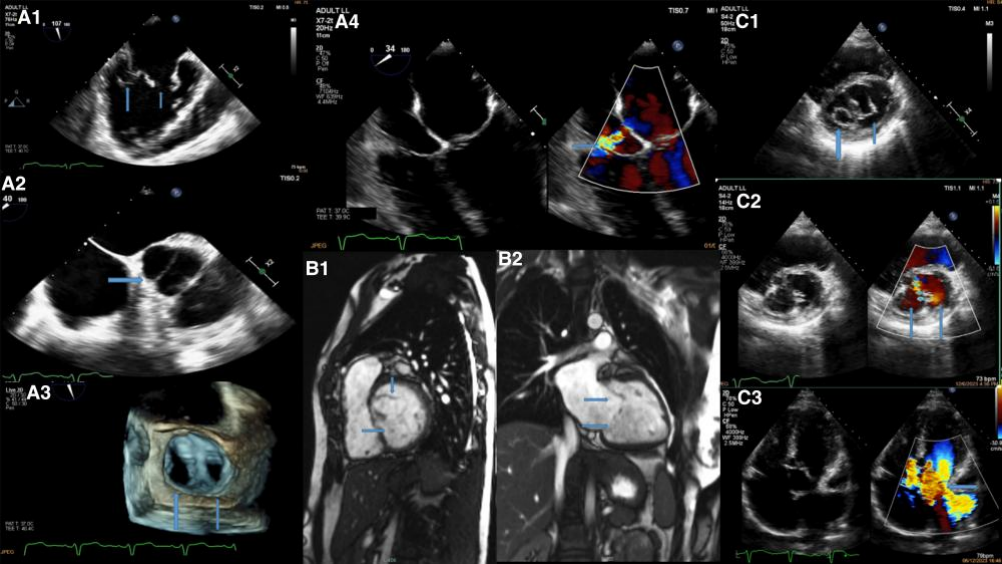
A transthoracic echocardiogram showed preserved left ventricular systolic function, biatrial dilation, bicuspid aortic valve with moderate regurgitation, DOMV with severe MR due to hyperdynamic conditions (pregnancy), and a dilated right ventricle with normal systolic function (*Panel C1–C3*; Supplementary material online, *Video S4*).
